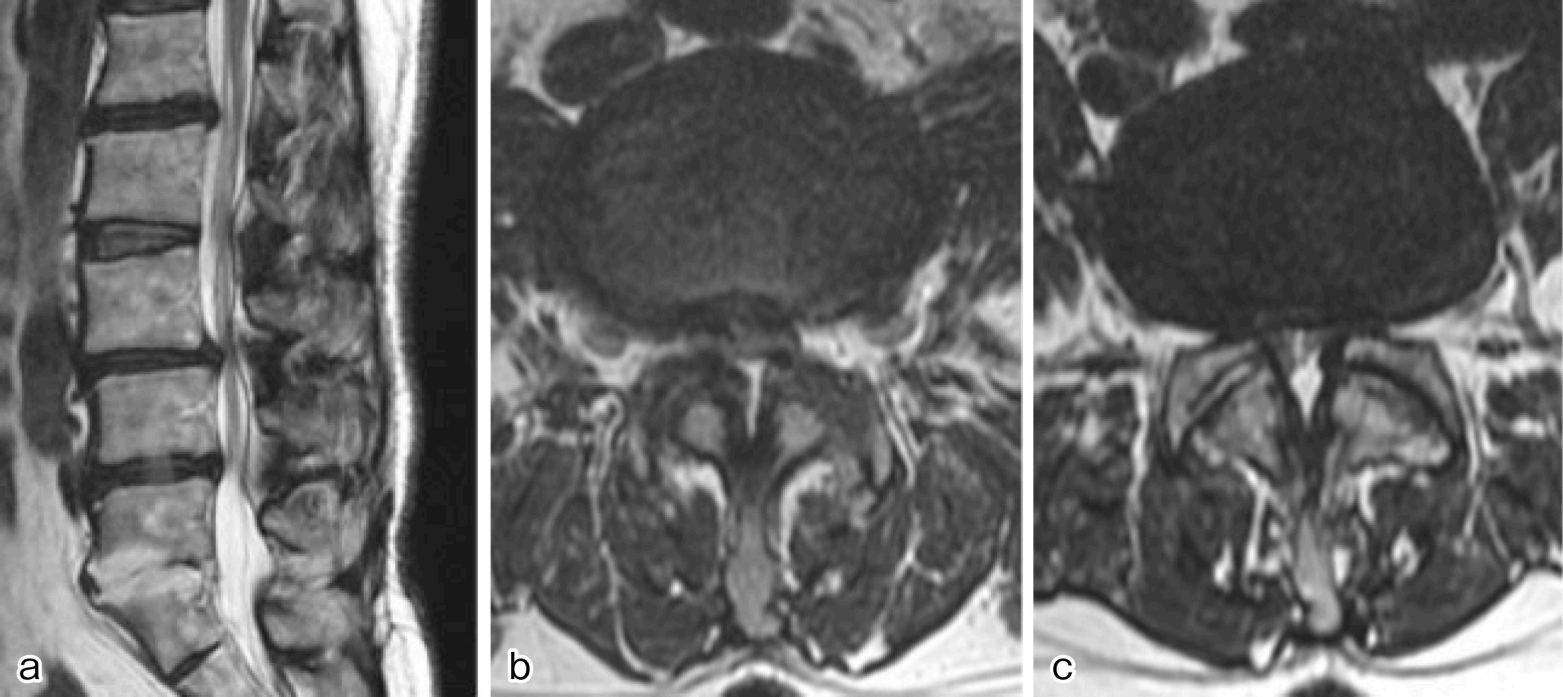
Preoperative magnetic resonance imaging T2-weighted images revealed severe spinal canal stenosis at L3-5. (a) Sagittal section, (b) horizontal section at L3-4, and (c) horizontal section at L4-5.
